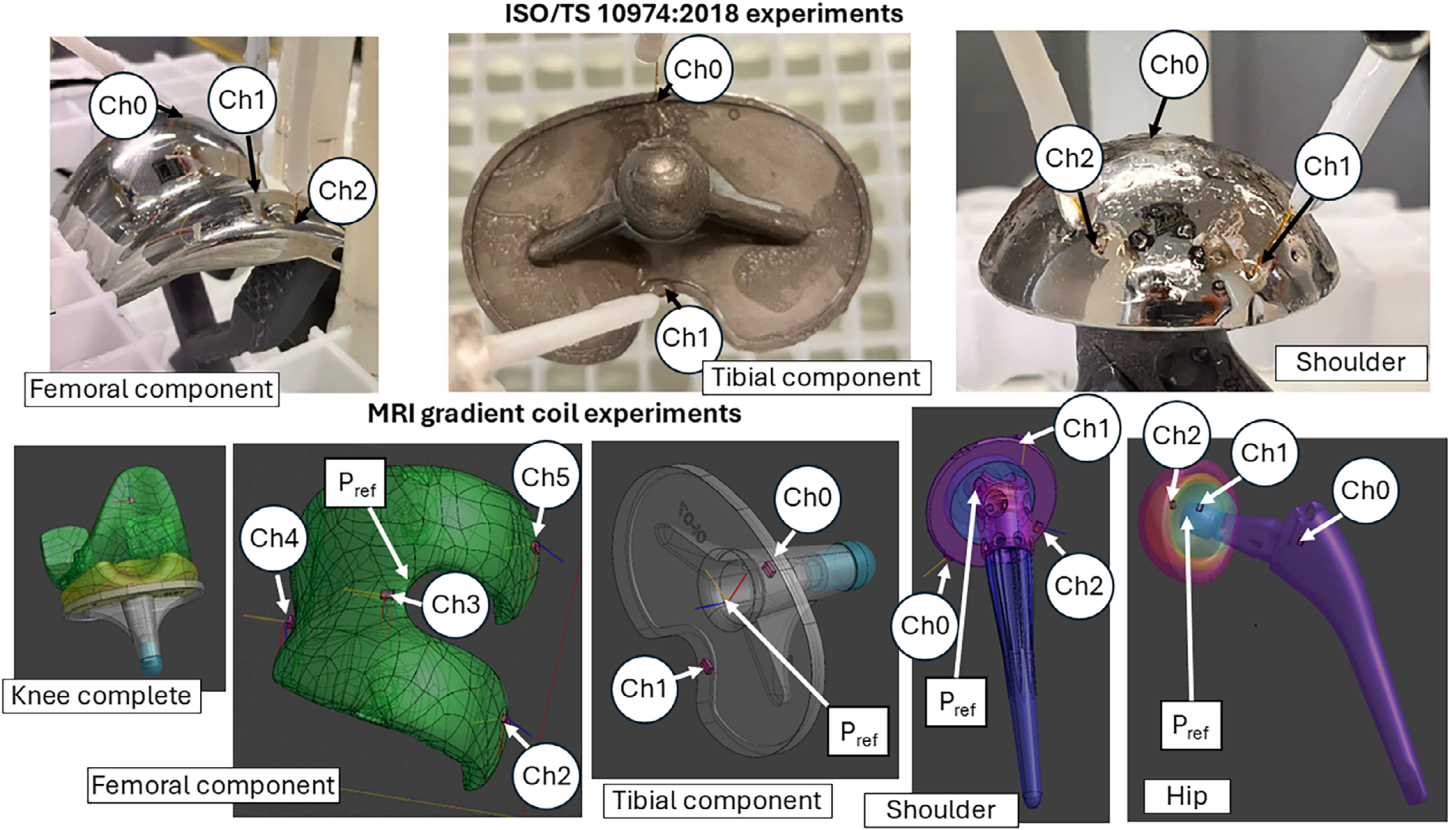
The top row shows photographs of the implants involved in the ISO 10974 experiments with the position of the thermal sensors indicated with labels. The bottom row shows the CAD models of the implants used in the experiments inside the MRI gradient coils. Also here the position of the thermal sensors is indicated with labels. The point P_ref_ is used as a reference to locate each model in a given position within the gradient coil system. Note that the thermal sensor labeling in the ISO/TS 10974:2018 experiments is different from the one used in the MRI gradient coil experiments.
